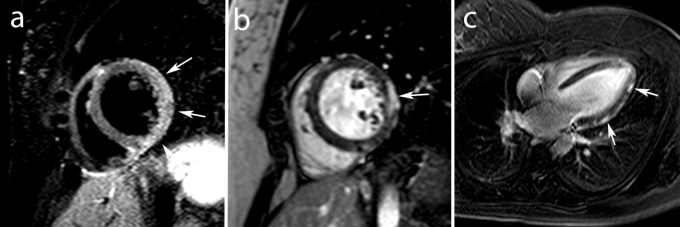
MRI study in an 18 year old male with acute chest pain in which acute myocarditis was diagnosed. Mid ventricular short axis T2–weighted STIR image shows edema (increased signal intensities) in the lateral wall (*arrows*, a) and short and vertical long–axis ce–MRI show typical subepicardial enhancement in the lateral wall (*arrows*, b,c).
